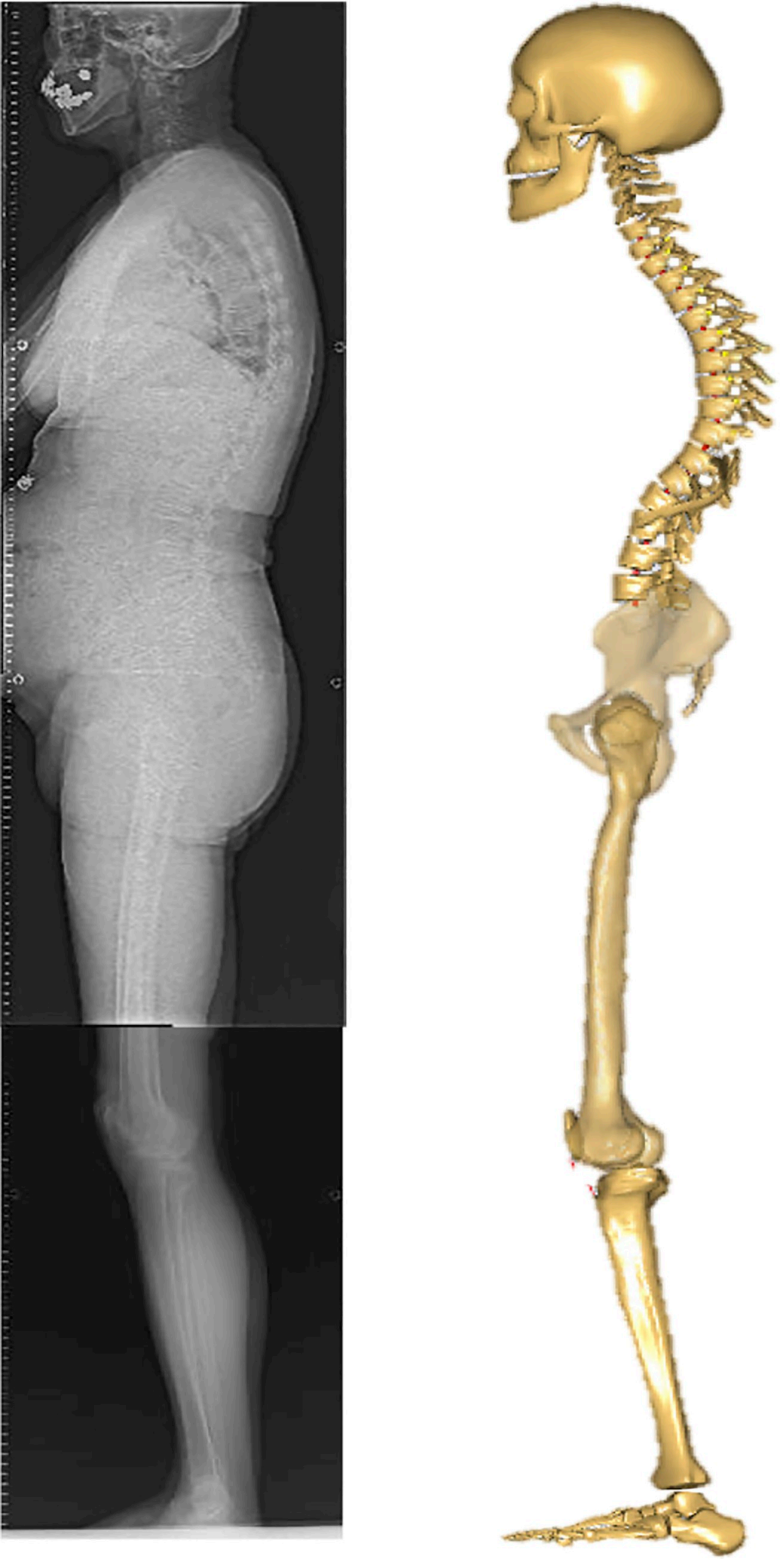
A patient’s full body lateral radiograph and images of the modified musculoskeletal model. This image shows the full-body lateral radiograph of a patient. A modified musculoskeletal model was used to input the patient’s sagittal alignment.
